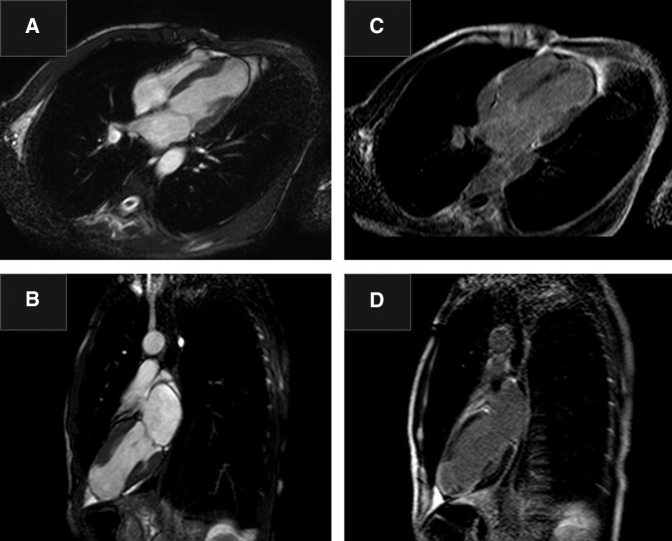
A, and B, Cardiac magnetic resonance imaging. C, and D, Delayed enhanced images.
